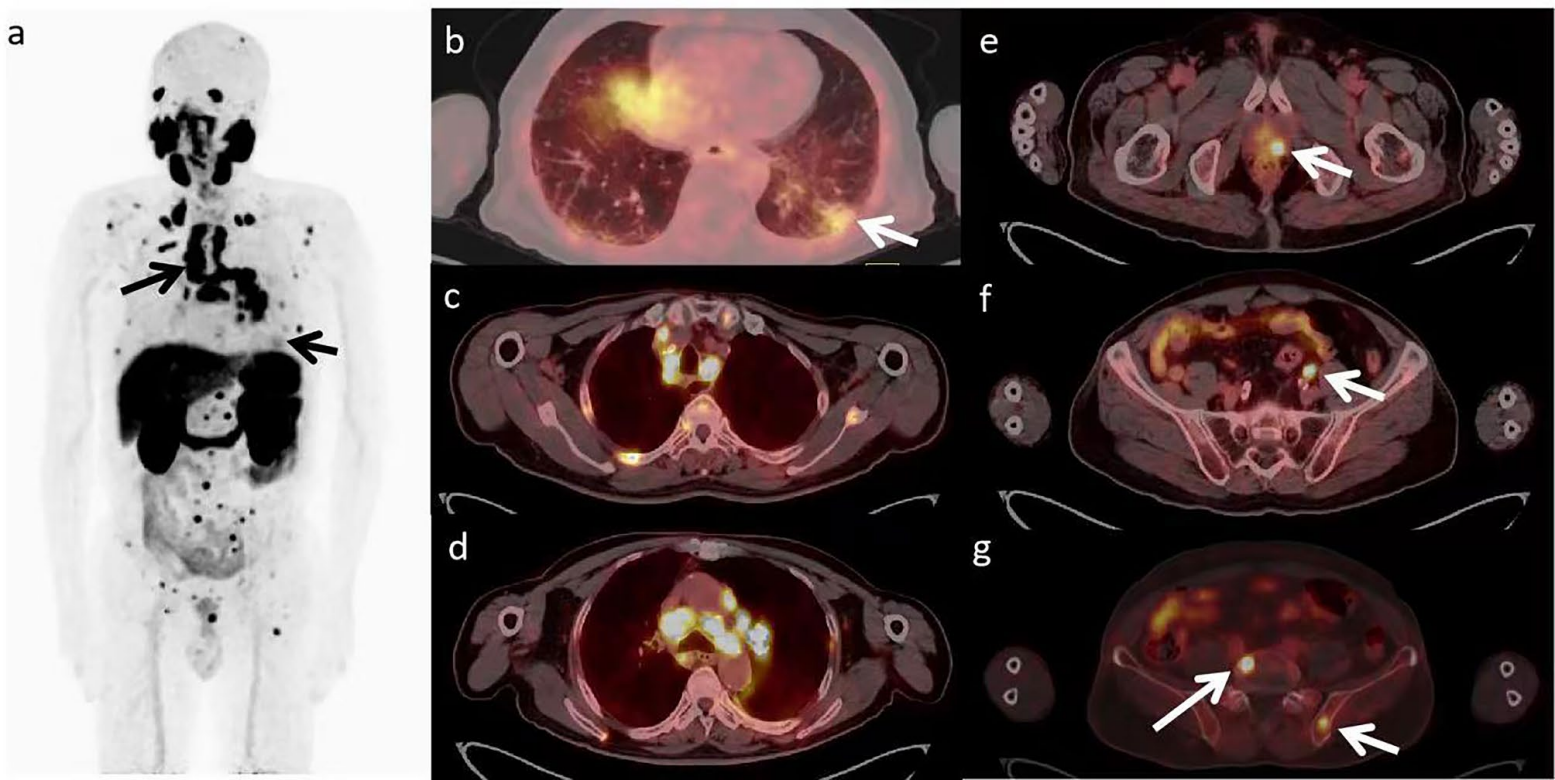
^18^ F-PSMA-1007 PET/CT images. a MIP image showed a increased PSMA uptake in left lobe nodule of prostate, left iliac lymph node and bone lesion. Meanwhile, the left pulmonary nodule and mediastinal lymph nodes had different degrees of PSMA uptake (arrows). b Axial PET/CT fused image showed relatively lower metabolism in left pulmonary nodule, SUVmax 5.1 (arrow). c,d Hypermetabolic multiple mediastinal metastatic lymph nodes, SUVmax 13.2–17.4. e Hypermetabolic nodule in left lobe of prostate, SUVmax 19.1 (arrow). f Hypermetabolic left iliac lymph node, SUVmax 18.5 (arrow). g Hypermetabolic metastasis in L5 vertebra and pelvic bone (arrows), SUVmax 17.0-36.9
